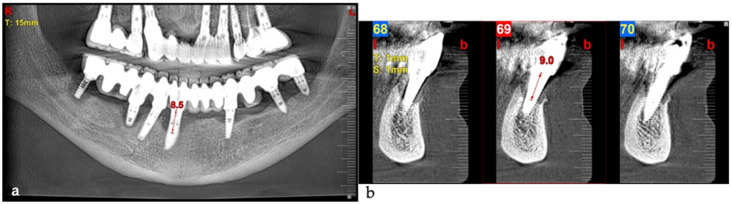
Panoramic radiographic view (a) and cone-beam computed tomography (CBCT) (b), revealing bone loss at baseline at tooth #41, respectively, 8.5 mm and 9.0 mm (red arrows).
